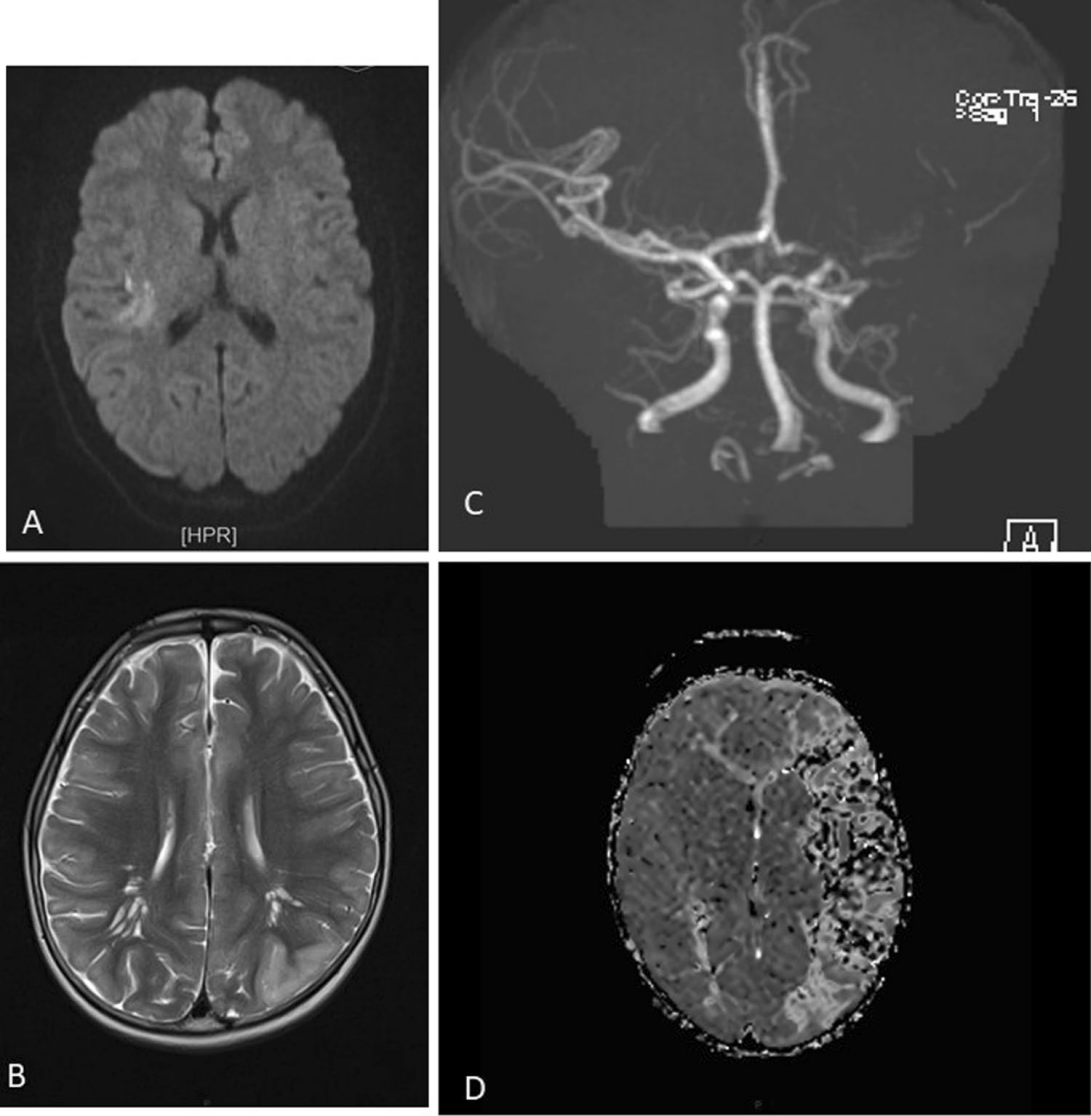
A Right parietal FLAIR-hyperintense lesion in a 14-year-old girl who presented with a complete anaesthesia of the left forearm and hand, visual impairment and headache. B Left temporo-occipital T2-lesion in a 6-year-old boy who presented with aphasia. C and D Angio-MRI and PWI sequences in a 2-year-old boy with the occlusion of M1 tract of the left-middle cerebral artery and of the A1 tract of the ipsilateral anterior cerebral artery
